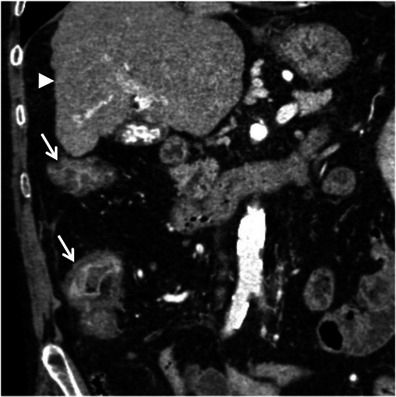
Stratified attenuation pattern in the ascending colon of a cirrhotic patient. Reformatted coronal contrast-enhanced CT scan shows parietal regular and concentric thickening of the ascending colon (*arrows*) with a “target” appearance due to oedema of the submucosa. Note the irregular contours of the liver (*arrowheads*) consistent with hepatic cirrhosis. *Adapted from the electronic poster “Bowel wall thickening—a complex subject made simple” DOI:*10.5444/esgar2011/EE-063
